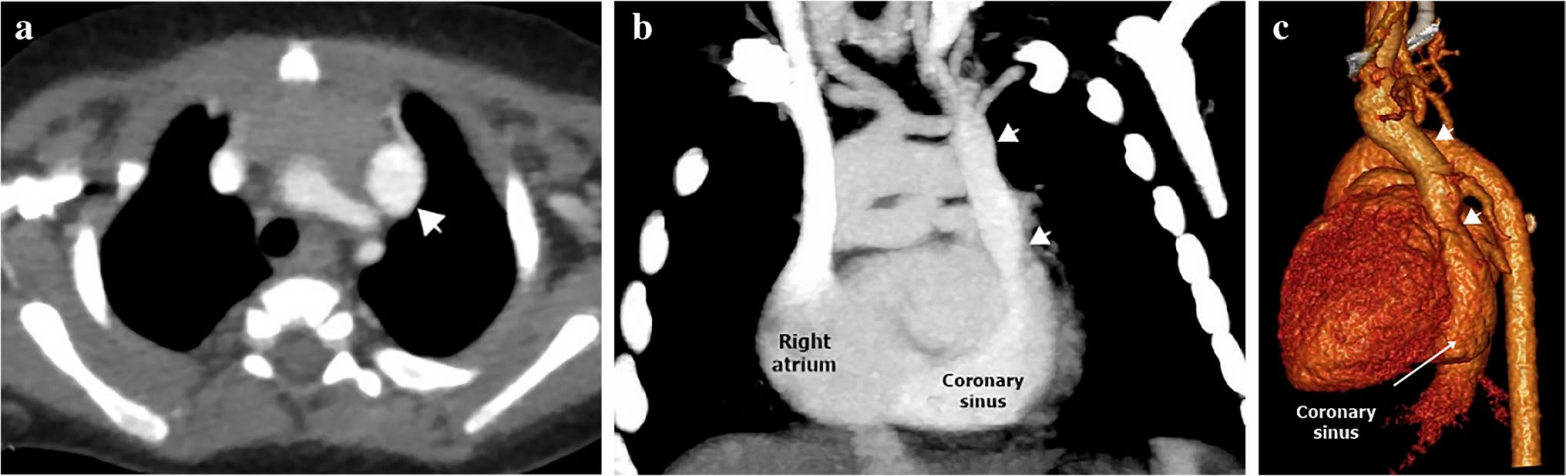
A persistent left superior vena cava (SVC) with no other anomalies in a 5-month-old boy. (a) An axial computed tomography (CT) image shows the persistent left SVC *(arrow)* on the left side of the mediastinum lateral to the aortic arch branches. b, c Coronal oblique CT image (b) and volume-rendered CT image (c) show drainage of the persistent left SVC *(arrows)* into the right atrium via the coronary sinus
